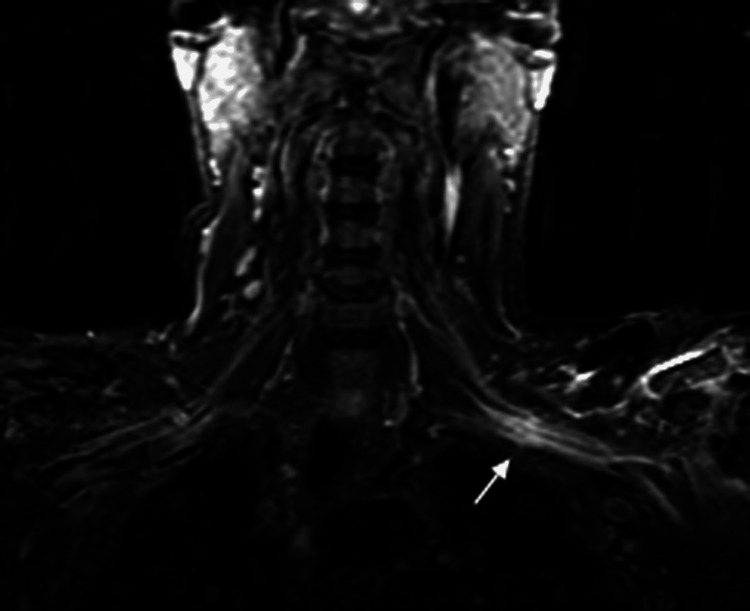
Coronal T2-STIR MR image of the patient’s left shoulder. The arrow shows enhancement of the left brachial plexus. Because of this diffuse signal abnormality, the neurosurgeon consultants opined that the patient’s deficits were predominantly inflammatory in nature and consistent with PTS. Therefore, additional surgery was not recommended, as it was believed that further surgical manipulation or dissection could worsen the patient’s deficits. PTS: Parsonage-Turner syndrome; T2-STIR: T2-weighted Short Tau Inversion Recovery.
